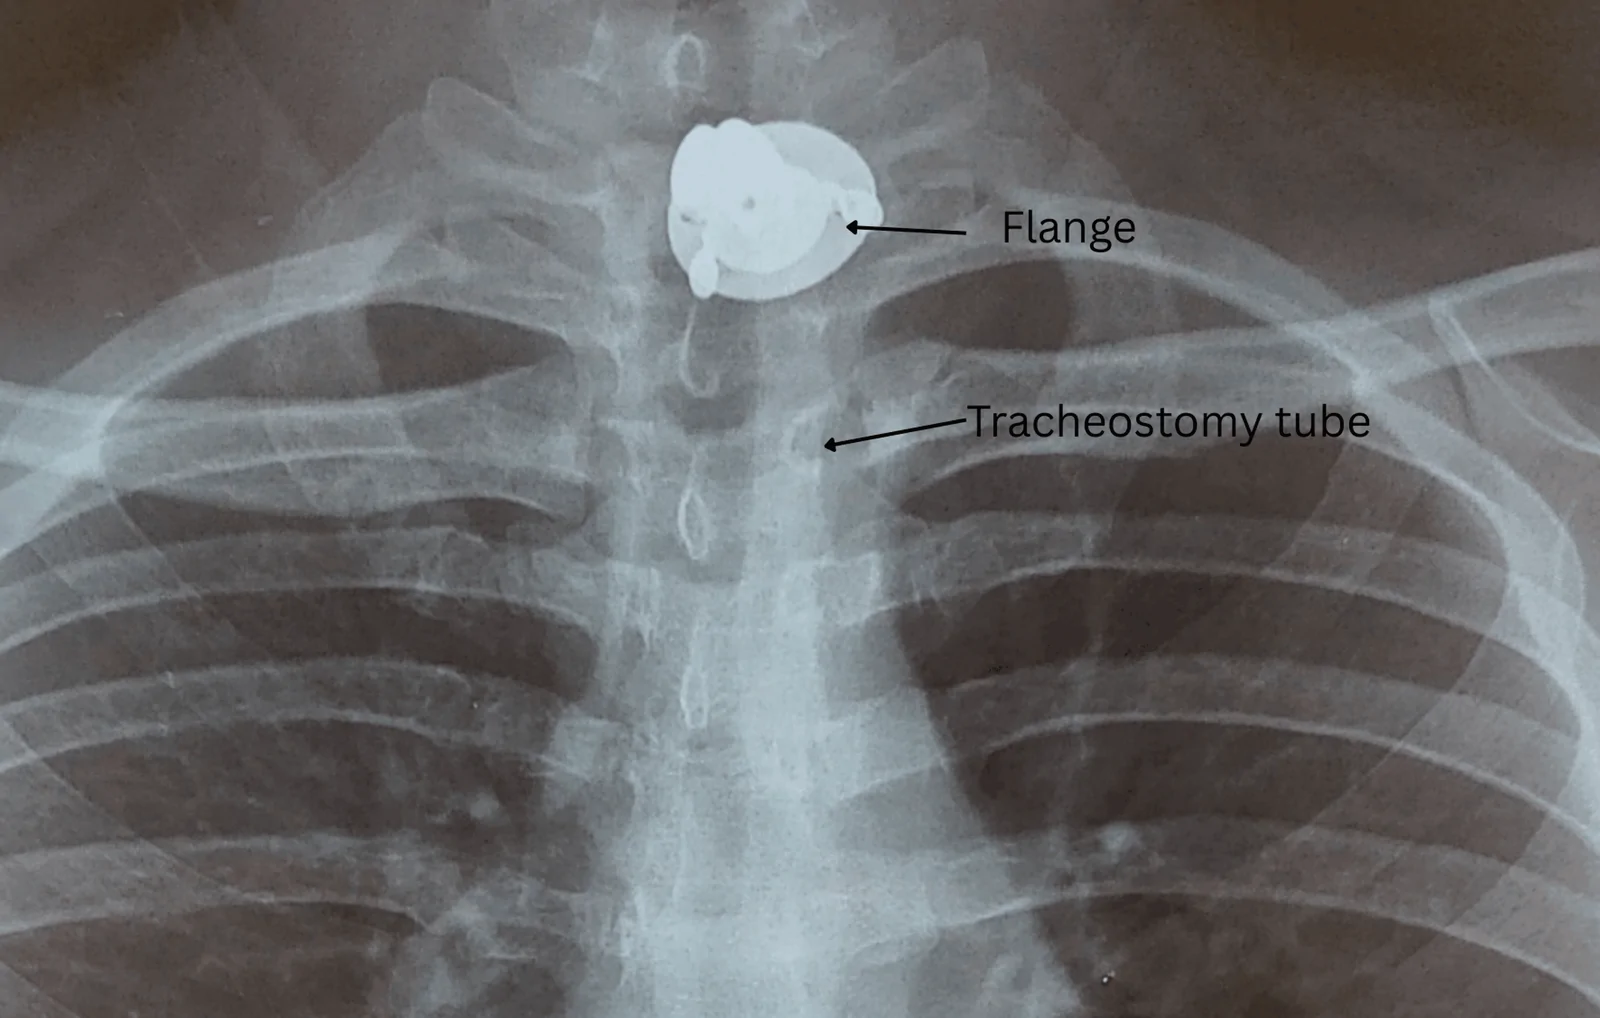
Chest X-ray (anterior view) done after tracheostomy confirming position
